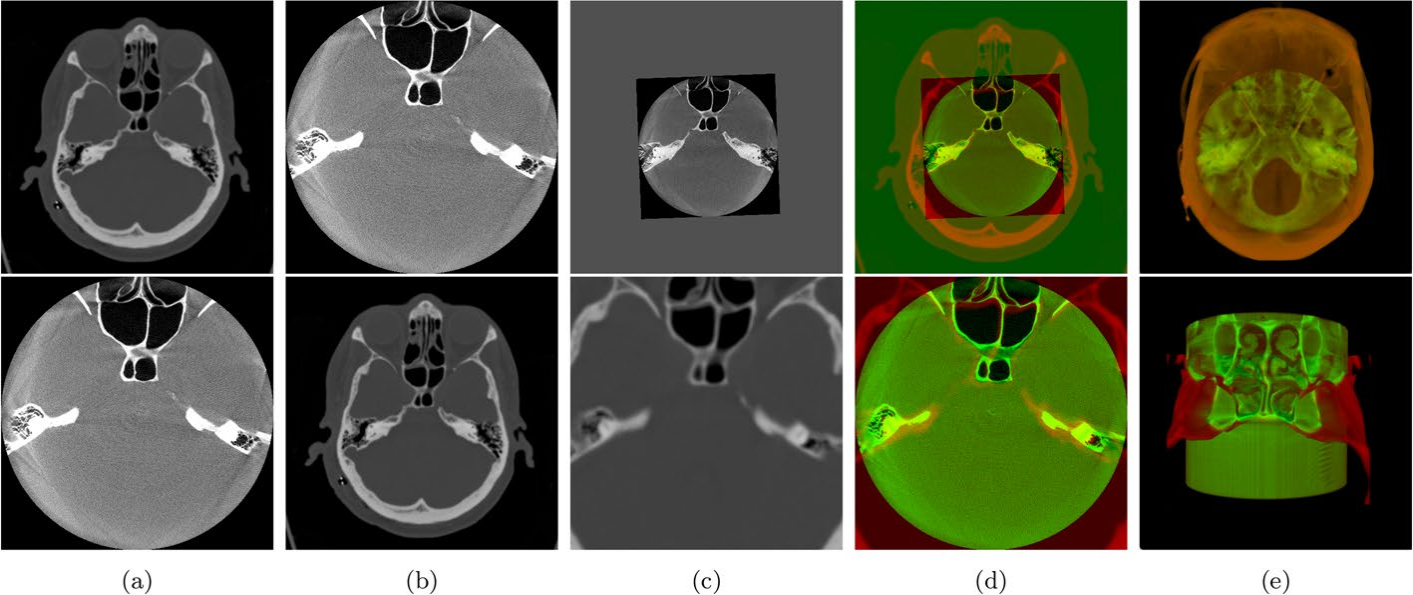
Representative images for a patient’s CT and DCTm in unregistered, registered, and fused form: a fixed images, b moving images, c registered images, d fused images, and e 3D fused images
